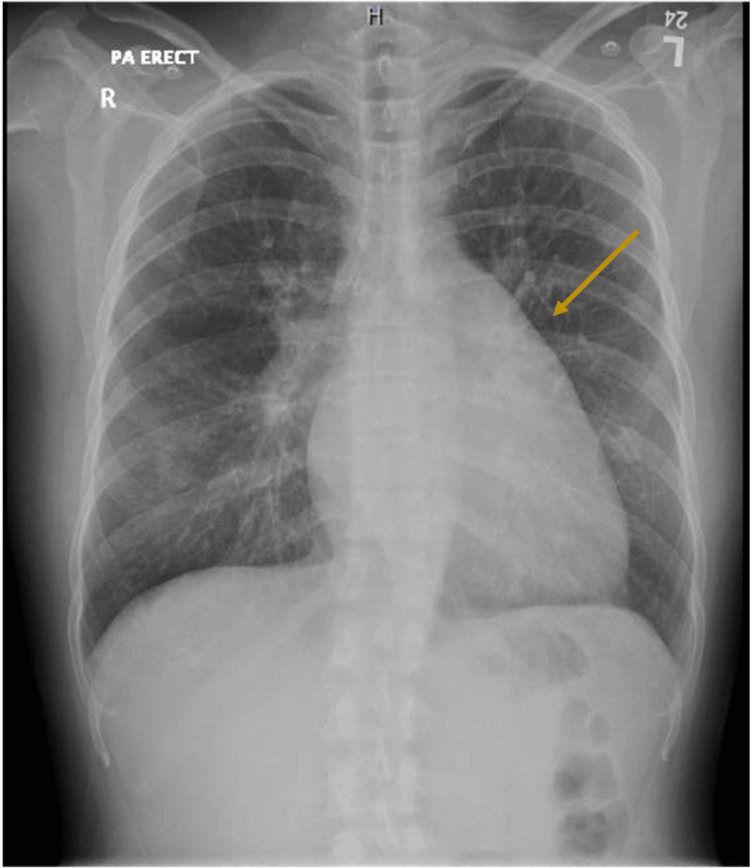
Chest X-ray on her first admission
